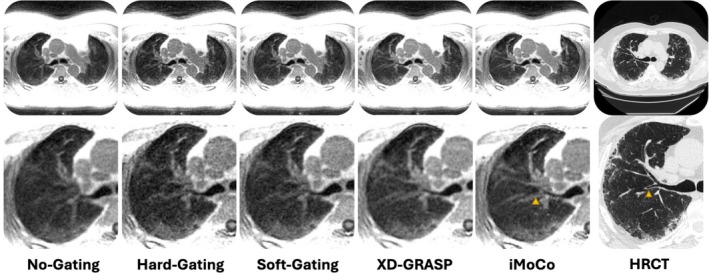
Representative slice from a patient diagnosed with IPF, with “possible UIP” pattern on CT, demonstrating visually evident improvement with advanced motion compensation schemes. An anatomically matched slice from high‐resolution CT is shown at the right for structural reference. The top row shows the complete slice, while the bottom row shows a closer view of the right lung to highlight differences. The orange arrow indicates location of an airway segment visible on CT, that can be identified using iMoCo reconstructions, but is obscured in other schemes. Fibrotic changes in the posterior lung are evident across all schemes to varying extents.
